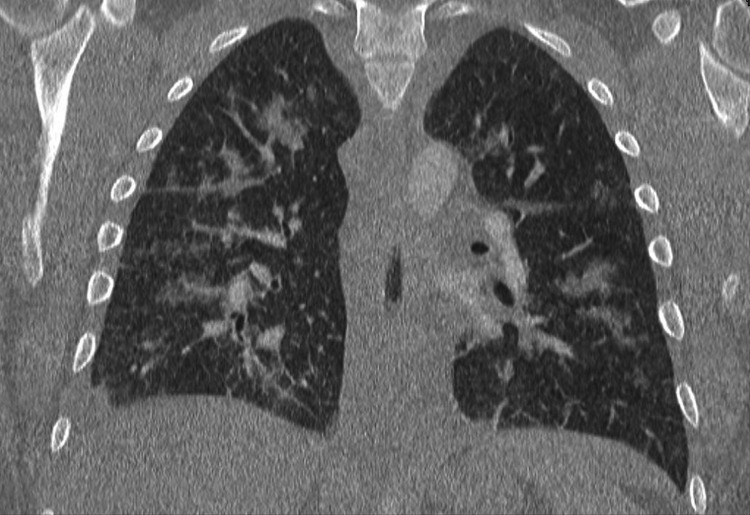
Chest computed tomography (CT) scan with contrast Bilateral perihilar and peribronchial cuffing, with enlarged mediastinal lymph nodes.
